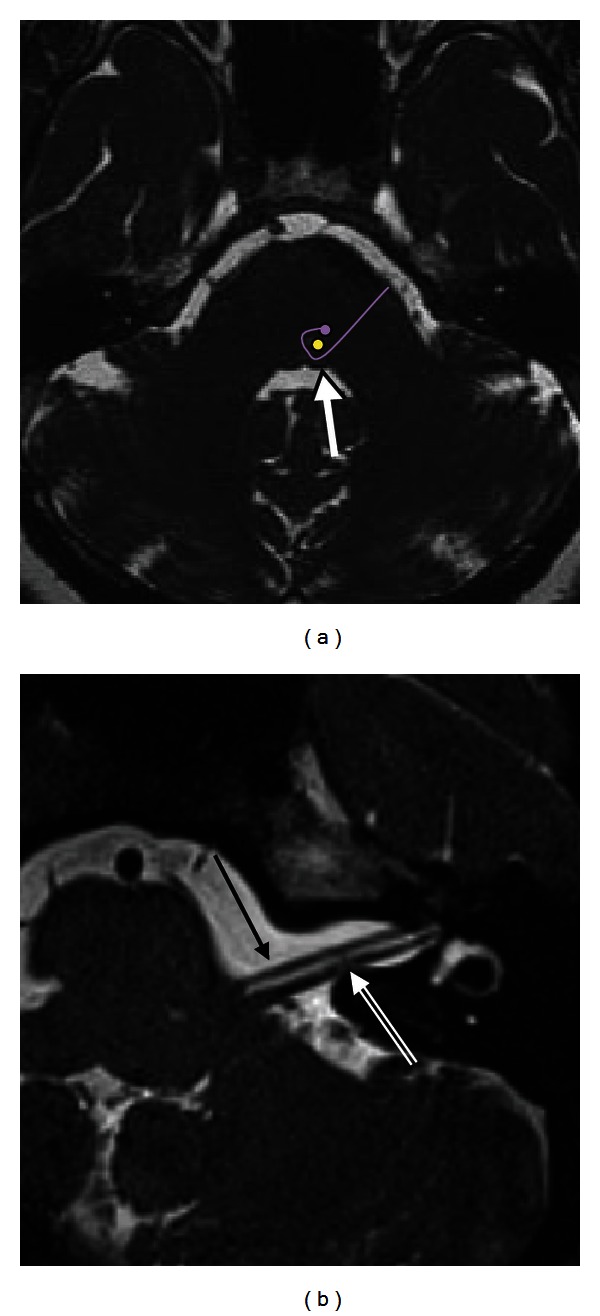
Normal facial nerve on MRI. (a) Axial CISS image at the level of the pons demonstrates the facial colliculus (arrow) seen as a small bump along the anterior wall of the fourth ventricle. This is formed by the motor tracts of the facial nerve (purple curved line) coursing around the abducens nucleus (yellow dot). (b) Axial CISS sequence of the left CPA and IAC demonstrates the normal cisternal and intracanalicular segments of the left CN VII (solid arrow), anterior to CN VIII (double lined arrow).
